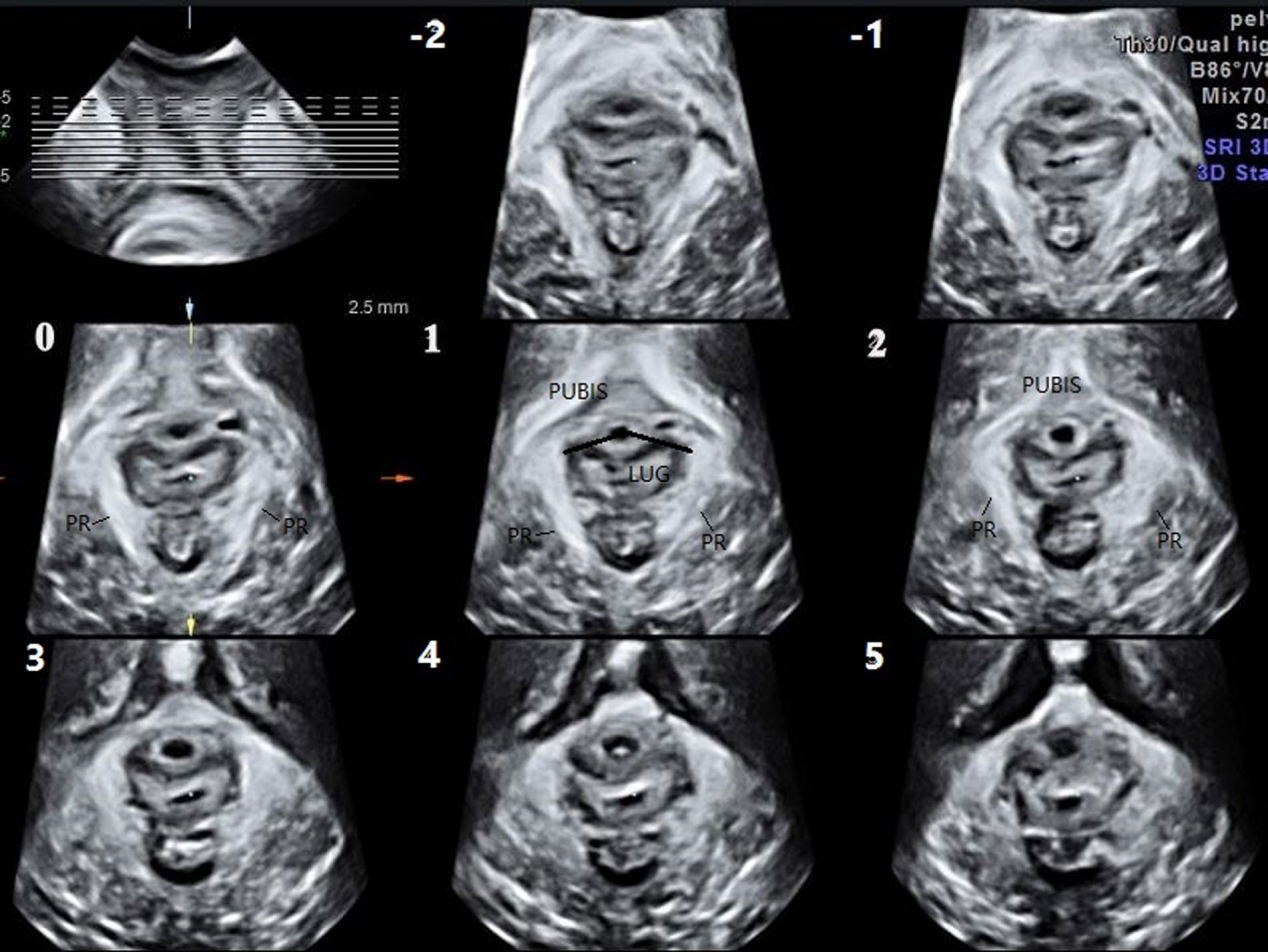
Images of the normal levator ani muscle of a 20-year-old nulliparous woman on TUI are presented. The image in the top left corner is a reference slice in the coronal plane. The other eight images represent axial plane slices obtained at 2.5-mm intervals; slice 1 is obtained in the plane of the minimum levator hiatus; the -1, -2, and 0 slices are caudal of the minimum plane; and the 2, 3, 4, and 5 slices are cephalad of the minimum plane. LUG was measured in slices 0, 1, and 2 and then averaged. (TUI: Tomographic ultrasound imaging; LUG: Levator-urethra gap, PR: puborectalis).
